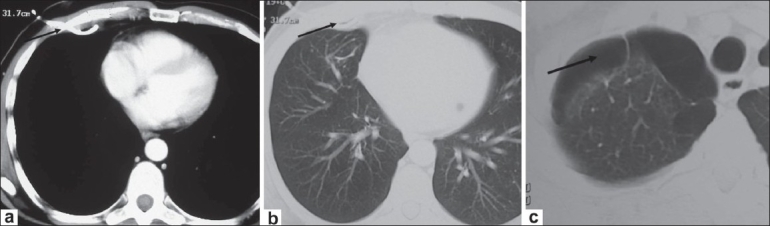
Post-drainage axial CT scan of the chest done two weeks after the pigtail drainage. Mediastinal (a) and lung window (b,c) images show near complete resolution of the air-fluid level with the pigtail catheter *in situ* (arrows in a and b) along with multiple bullae (arrow in c) in the apical segment of the right lung
